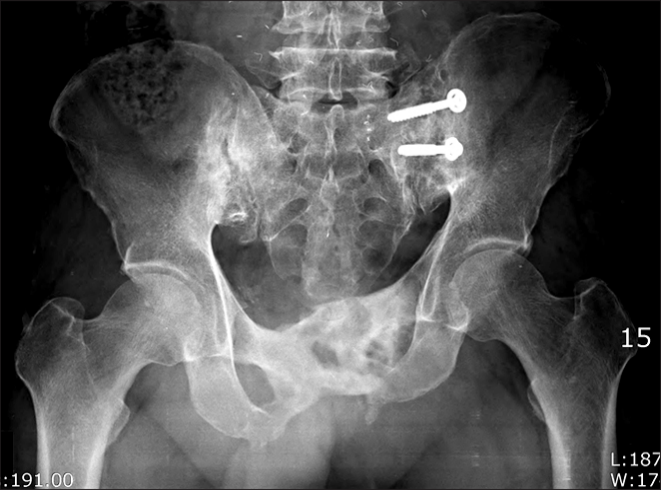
Initial anteroposterior radiograph of the pelvis.
